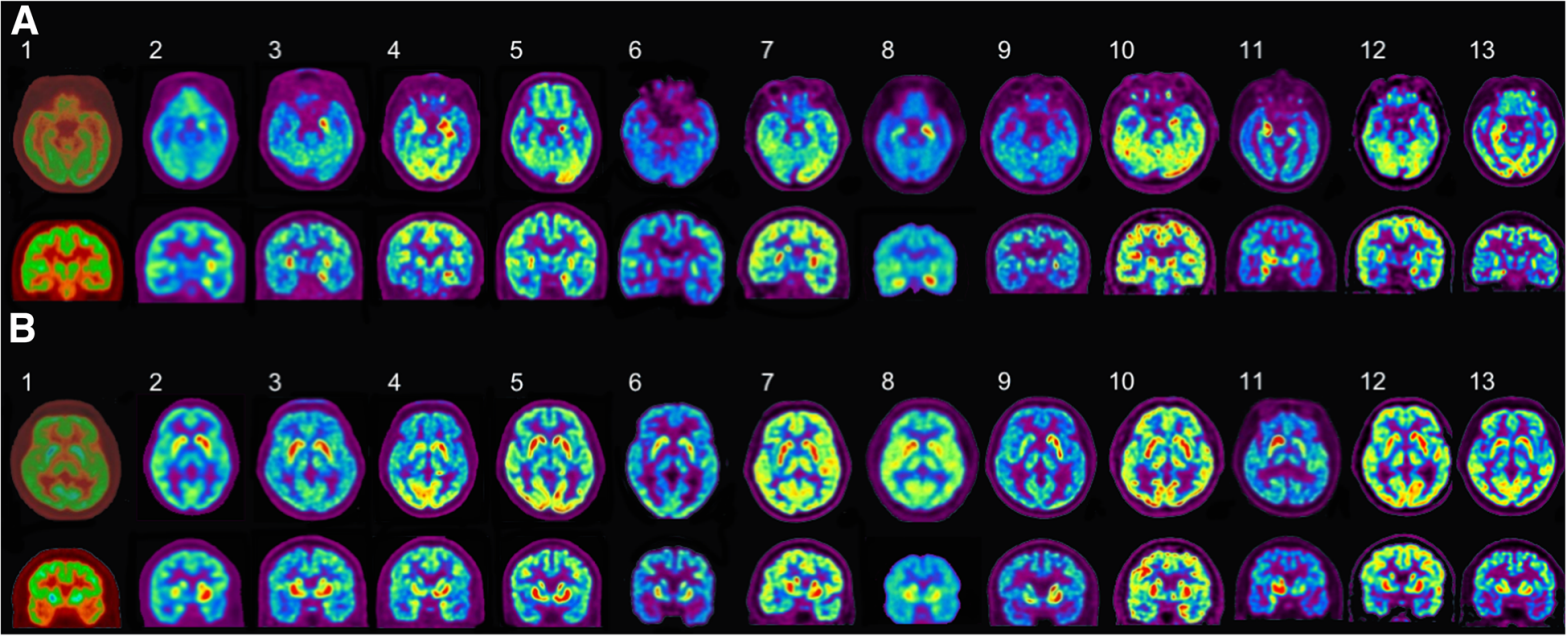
The pretreatment 18F-FDG-PET images of patients with LGI1-antibody encephalitis. a Axial (upper row) and coronal views (lower row) of the hippocampus: out of the 13 patients, nine (2, 3, 4, 5, 6, 7, 8, 10, and 12) had increased FDG uptake on the left side, two (11, 13) had on the right side, and two (1, 9) had no laterality. b Axial (upper row) and coronal views (lower row) of the basal ganglia: seven (1, 2, 4, 8, 9, 11, and 12) had asymmetric FDG uptake in the basal ganglia. Among them, four (2, 4, 9, and 12) had increase uptake on the left side, and three (1, 8, and 11) on the right side. All patients were right-handed except one (11), who was left-handed. R = right, L = left, 18F-FDG-PET = 18F-fluoro-2-deoxy-d-glucose positron emission tomography, LGI1 = leucine-rich glioma-inactivated 1
